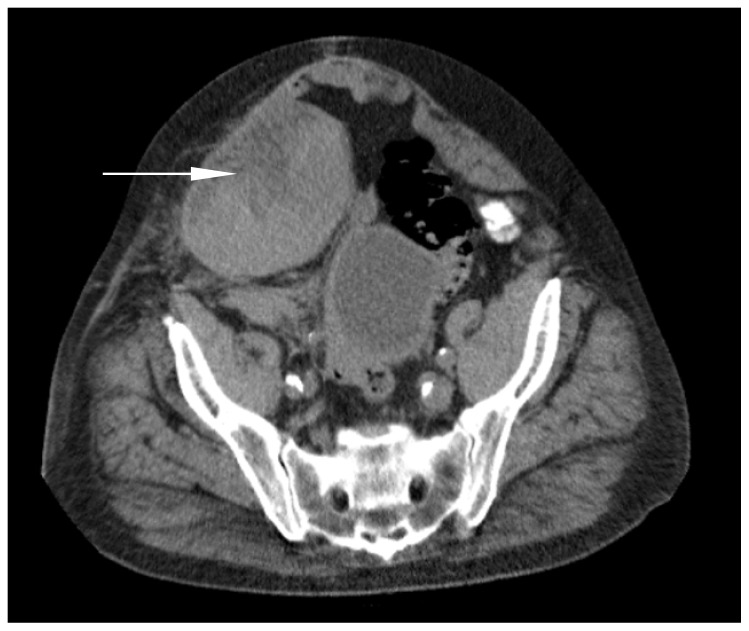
Axial view computed tomographic scan revealing hematoma within the rectus sheath (arrow).
